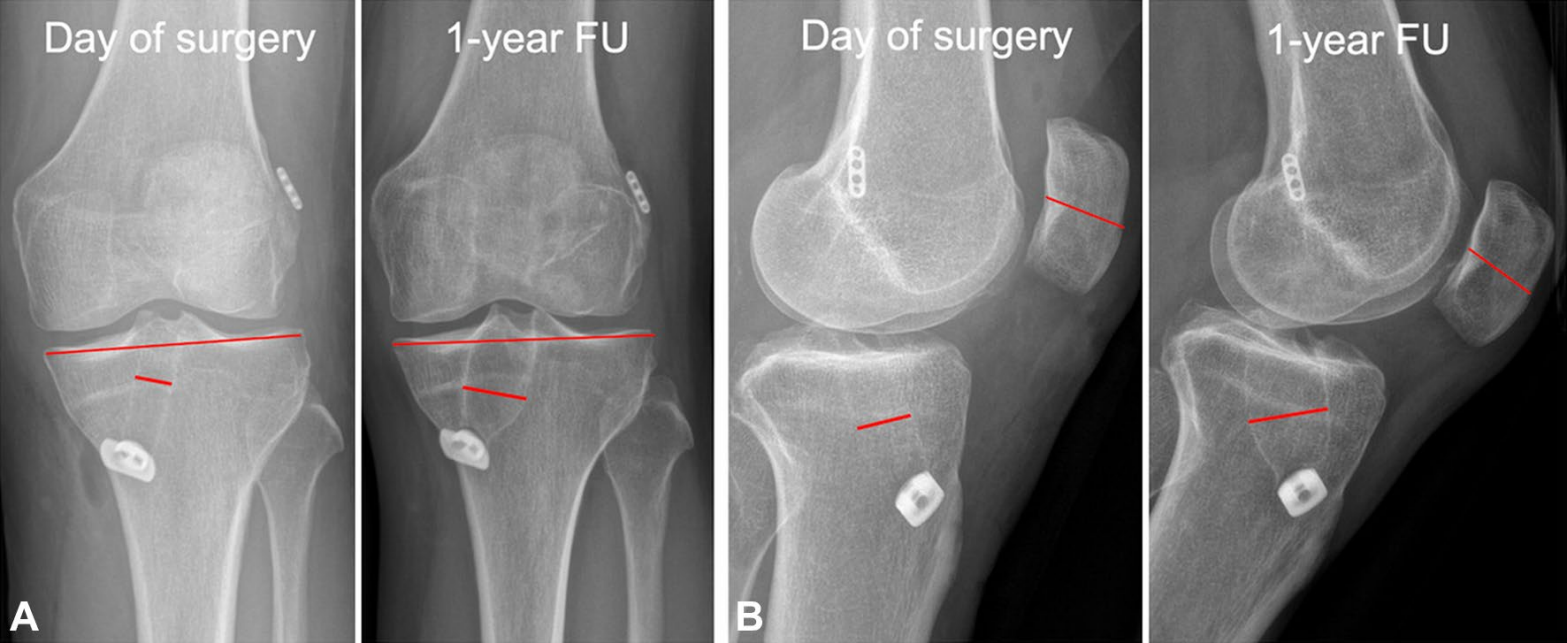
The sclerotic margins of the tibial bone tunnel were measured at the widest dimension of the tunnel in the frontal and sagittal plane, and compared to the first postoperative radiograph after correction for radiographic magnification. A percentage change between the two periods was defined as percentage tunnel enlargement in diameter, and a change of more than 10% was defined as an enlarged tunnel
